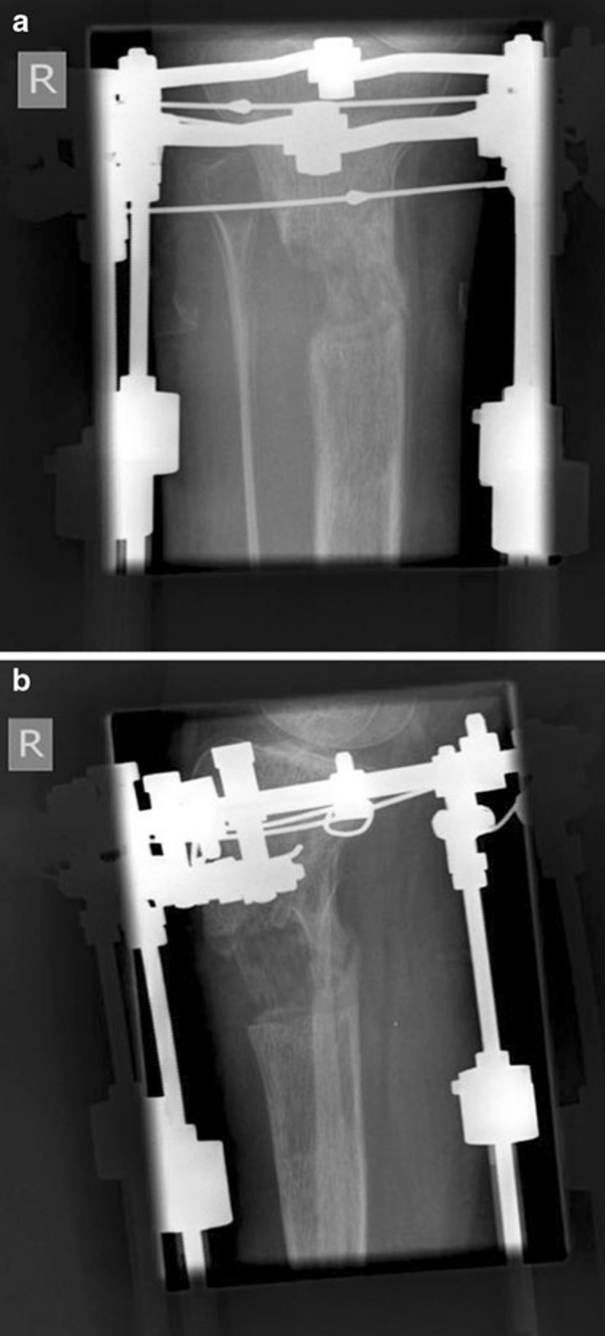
a Anteroposterior and b lateral radiographs of the proximal tibia 4 months into distraction osteogenesis showing “asymmetric scalloping” of the posterolateral aspect of the regenerate
